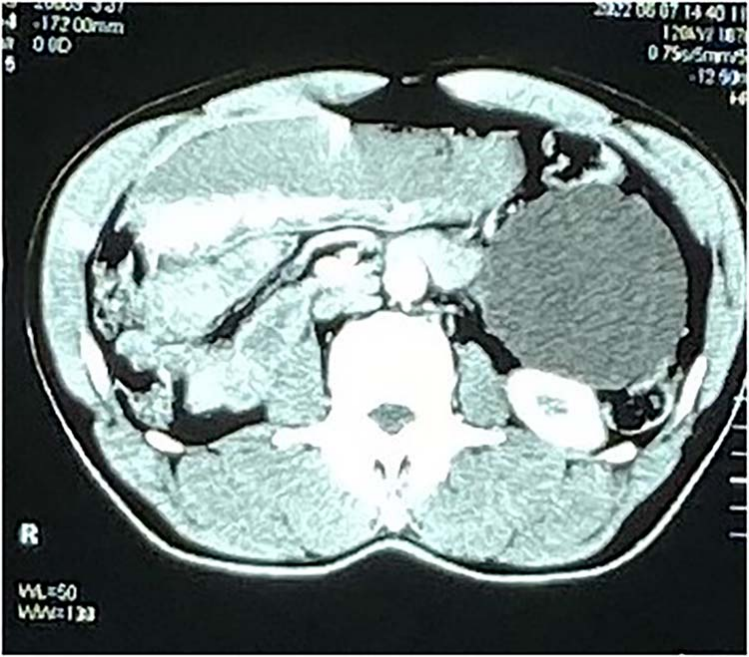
Axial CT image of the mesenteric cyst.
